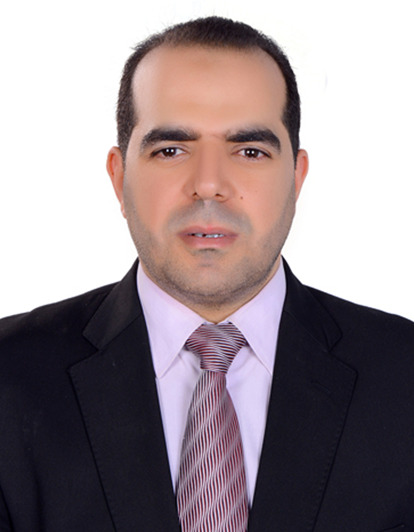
Dr Mohamed Sobh is a fellow in congenital cardiovascular magnetic resonance imaging (MRI) in the Department of Congenital Heart Disease and Paediatric Cardiology at the University Hospital Schleswig-Holstein, Campus Kiel. He has finished his training in paediatrics and paediatric cardiology in Egypt at the Cairo University. Currently, he focuses on clinical cardiovascular MRI imaging as well as on a research MRI project about the right ventricular function in patients with hypoplastic left heart syndrome.
